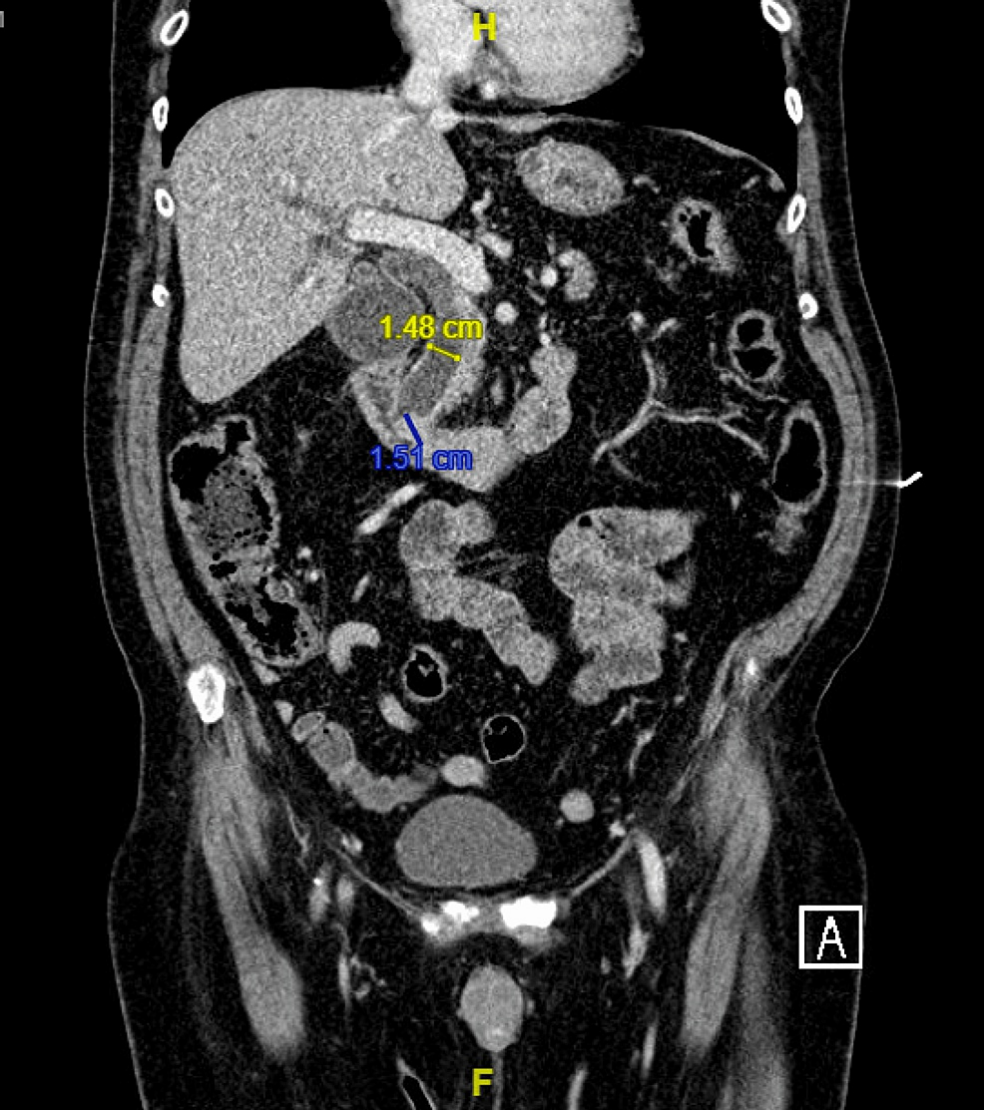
CT scan of abdomen and pelvis significant for 1.8 x 1.3 x 1.5 cm mass within the common bile duct resulting in marked bile duct dilatation
